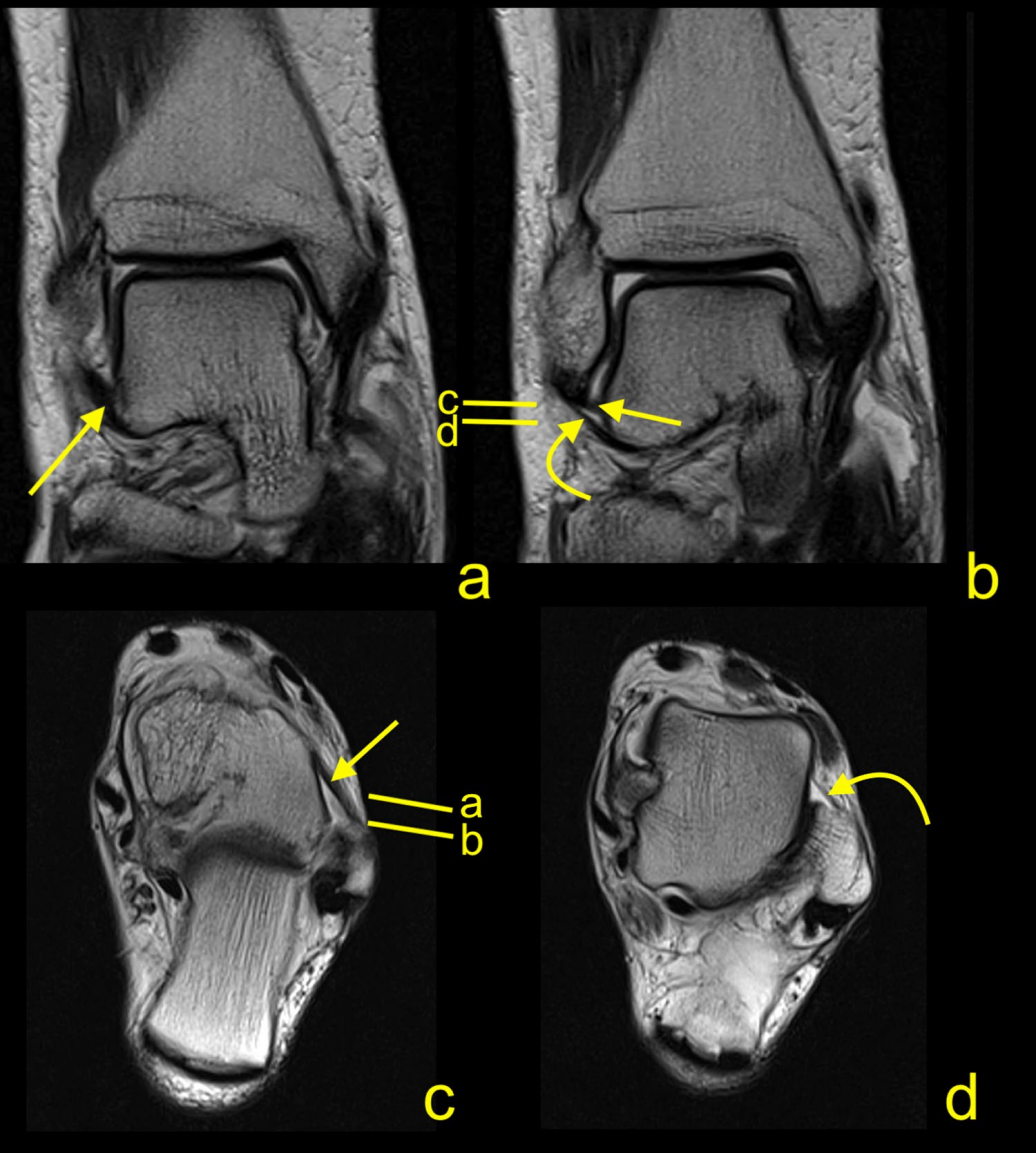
A 34-yeard-old patient with suspicion of the soft tissue tumor, which was not confirmed on the MRI. The double fascicular ATFL, two asymmetrical fascicles. Sagittal section (a,b) and transverse section (c). The S-ATFL (straight arrow), the I-ATFL (curved arrow), the PTFL (dashed arrow), and the CFL (curved dashed arrow). Levels of cross-sections are labeled on figures b and c.
